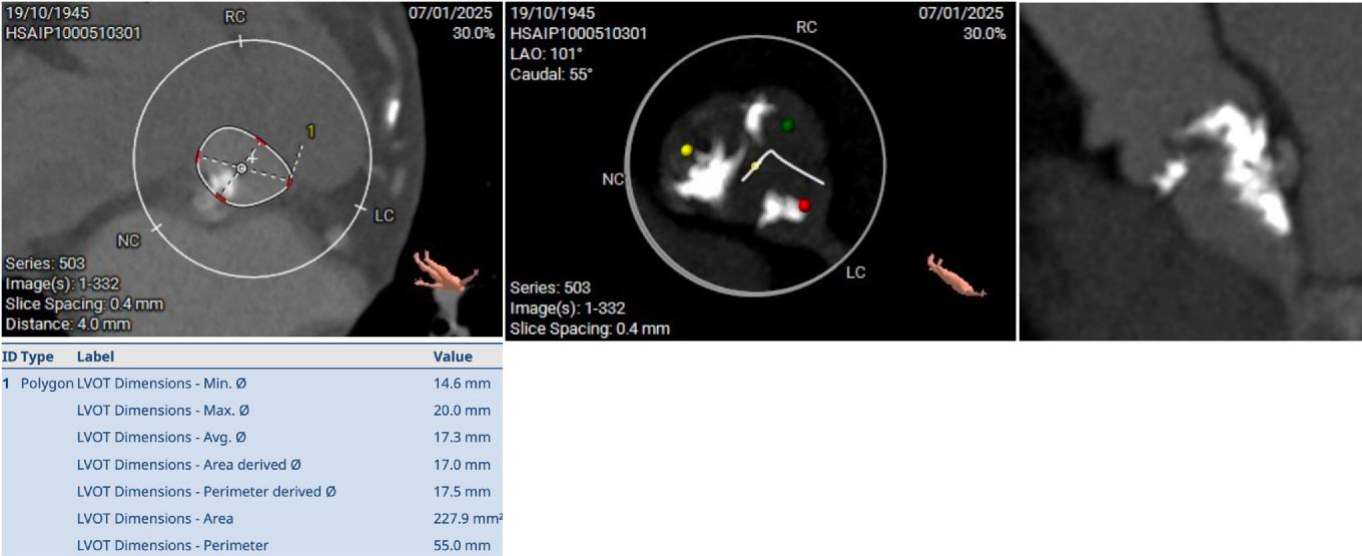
Preprocedural CT scan revealing the LVOT measurement and calcium burden.
